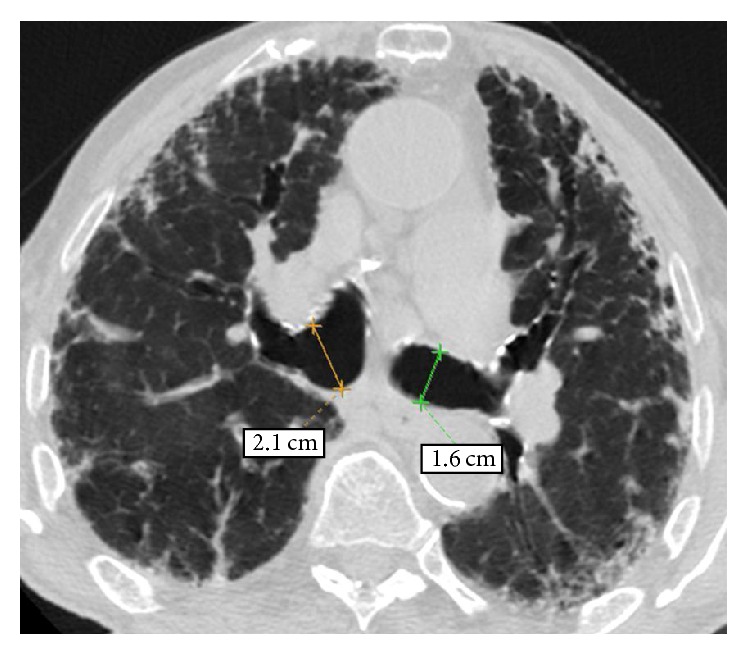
HRCT of the chest at level below carina shows dilated right and left main bronchi and undulating wall of left main bronchus, indicating bronchial diverticulosis. A subpleural reticular pattern associated with focal thickening of the pleura and traction bronchiectasis and bronchiolectasis is also noted.
